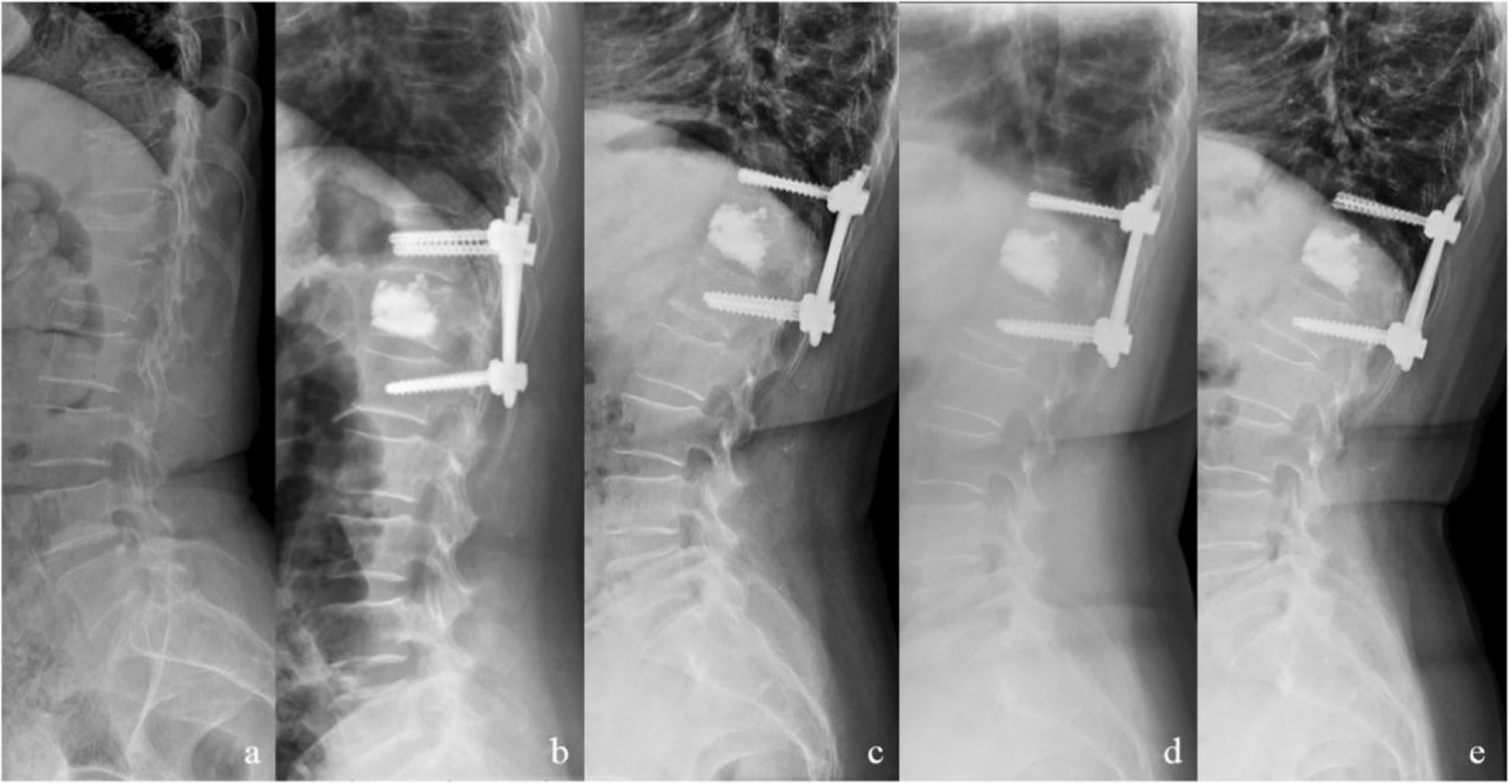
A 71-year-old female patient with osteoporotic compression fracture of T12 vertebral body with kyphosis was treated with PPSF combined with PVP. a T12 severe compression fracture with kyphosis in preoperative. b Postoperative 3D X-ray showed that the height of L1 vertebra recovered basically and kyphosis was corrected. c–e X-ray showed no significant loss of T12 vertebral body height and kyphosis in 6 months, 12 months, and 24 months follow-up
